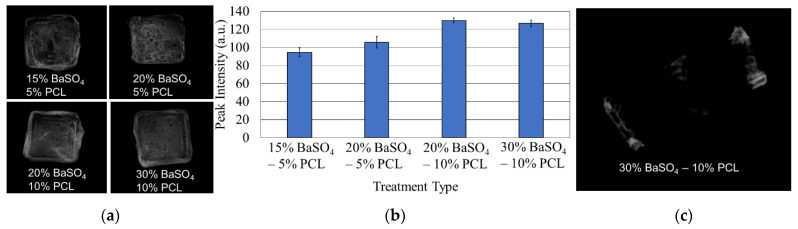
(a) X-ray images of flat PCL tiles coated with 15% BaSO_4_ and 5% PCL, 20% BaSO_4_ and 5% PCL, 20% BaSO_4_ and 10% PCL, and 30% BaSO_4_ and 10% PCL; (b) average peak intensity of coated PCL tiles with ± 1 standard error bars; and (c) PCL FDS ends coated with 30% BaSO_4_ and 10% PCL.
